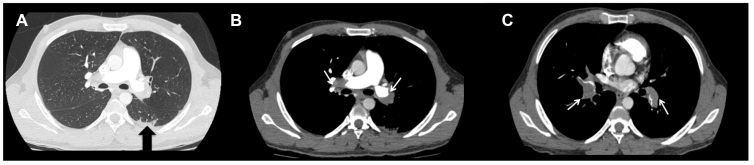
(A) Chest computed tomography, pulmonary window, axial, showing small areas of consolidation in the peripheral upper segment of the left lower lobe (black arrow), a typical finding in patients with COVID-19 pneumonia; (B and C) axial chest computed tomography with contrast in the mediastinal window. Large filling defects adherent to the walls of both the left and right main pulmonary arteries before their bifurcation (white arrows), an appearance compatible with pulmonary thromboembolism.
